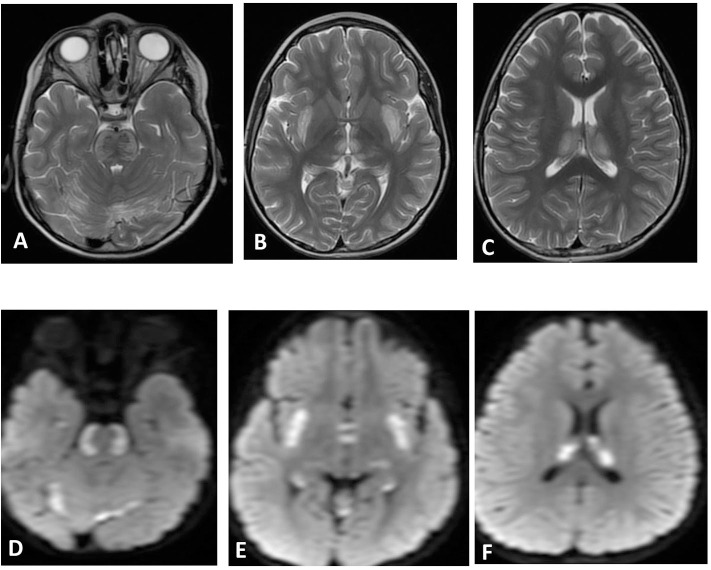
Brain MRI. Axial T2 (A–C) and diffusion (D–F) showing swelling and abnormal high signal intensity of pons, bilateral external capsule, and subinsular cortex, as well as both thalami with diffusion restriction.
